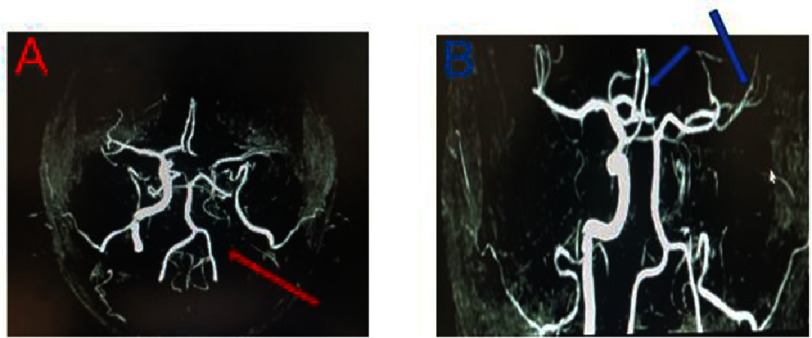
Angio-MRI of the supra-aortic trunks showing an absence of flow at the ostium of the left internal carotid artery (A: red arrow) associated with a collateral flow from communicating arteries (B: blue arrows) resulting first in a complete and chronic occlusion.
